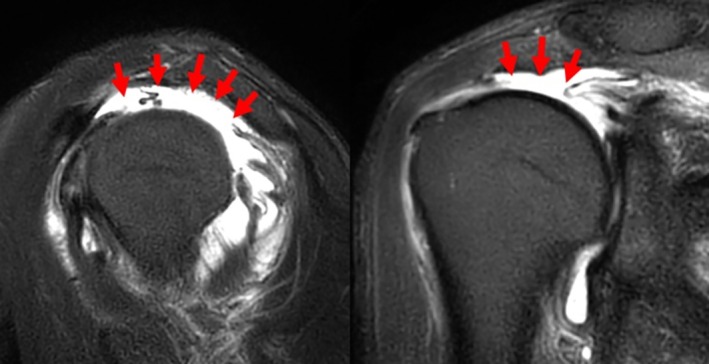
Magnetic resonance imaging at diagnosis showing a right rotator cuff tear (arrow).
